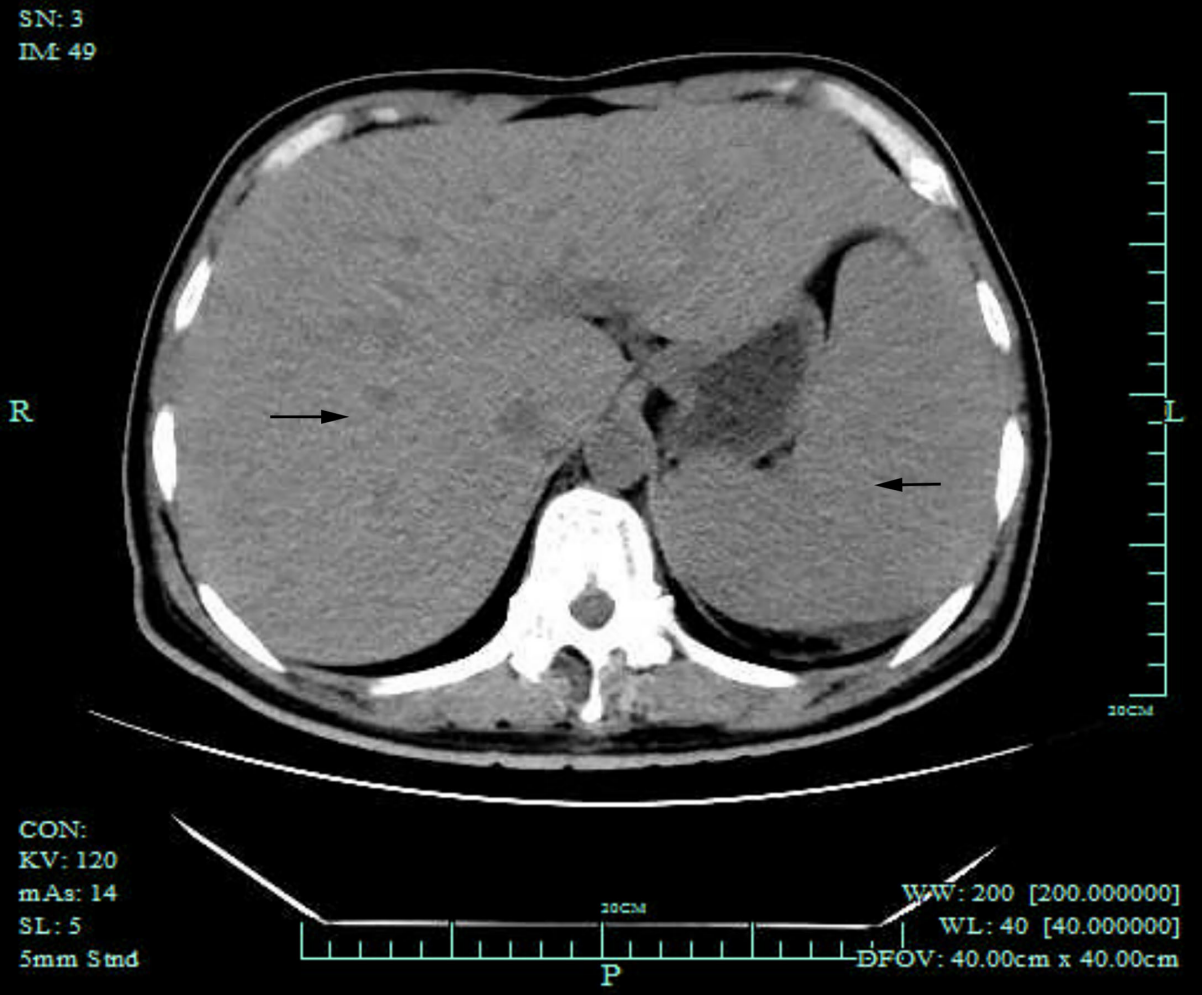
Abdominal CT scan showed splenomegaly and hepatomegaly (black arrows).
